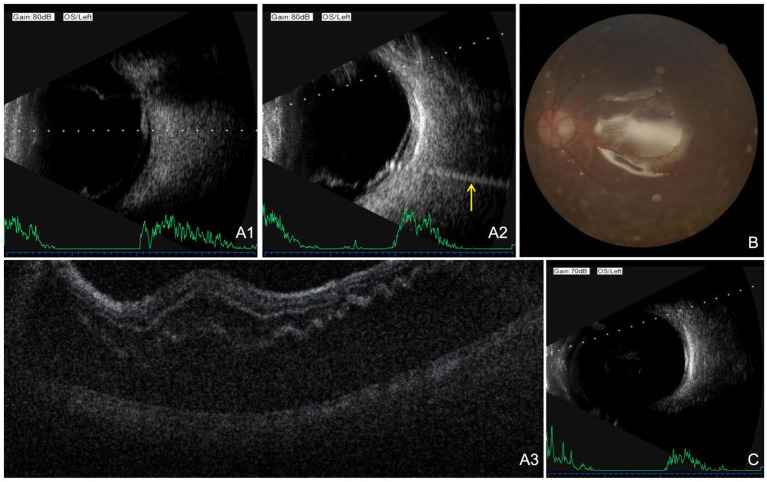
Multimodal imaging of rhegmatogenous retinal detachment (RRD) and outcomes after silicone oil removal of the left eye. (A1,A2) B-scan ultrasonography: Ultrasound revealed a V-shaped hyperechoic band in the vitreous cavity, attached to the optic disc, consistent with retinal detachment (yellow arrow: a linear acoustic artifact). (A3) Optical coherence tomography: The retinal neuroepithelium and the retinal pigment epithelium were separated in the scanned region. The internal architecture of the neuroepithelium was poorly defined, with visible undulating folds. (B) Color fundus photography: Silicone oil occupied the vitreous cavity, forming a well-defined reflective interface, with the retina remaining flat. (C) B-scan ultrasonography: Follow-up B-scan after silicone oil removal. Several highly echogenic silicone oil droplets were detected in the vitreous cavity, and the retina remained well attached.
